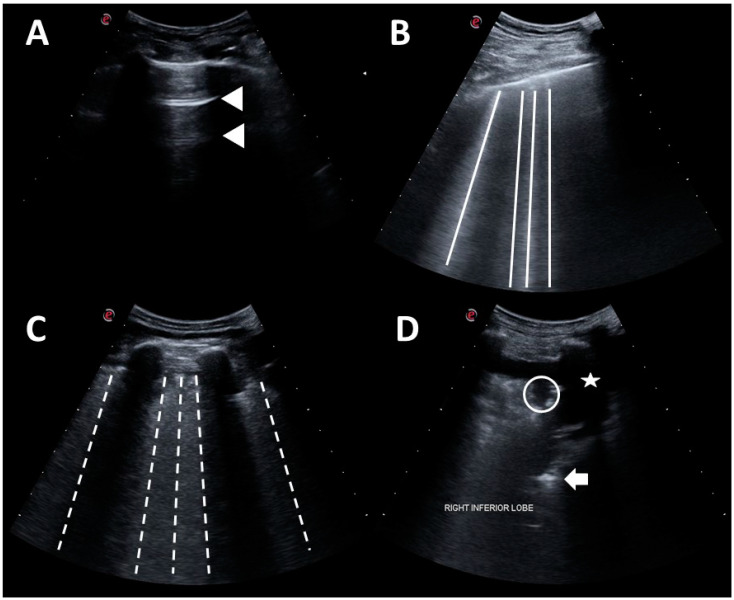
Lung Ultrasound Score was evaluated according to guidelines. (A) Score 0: normal pattern, A-lines or <3 B-lines; (B) Score 1: moderate loss, ≥3 B-lines; (C) Score 2: severe loss, coalescent B-lines; (D) Score 3: complete loss, white lung and/or lung consolidations. Legend: A-lines are indicated by triangles; B-lines are indicated by continue lines; coalescent B-lines are indicated by dashed lines; consolidation is indicated by circles; aerial bronchogram is indicated by an arrow; pleural effusion is indicated by stars (as reported in ref. [4]).
